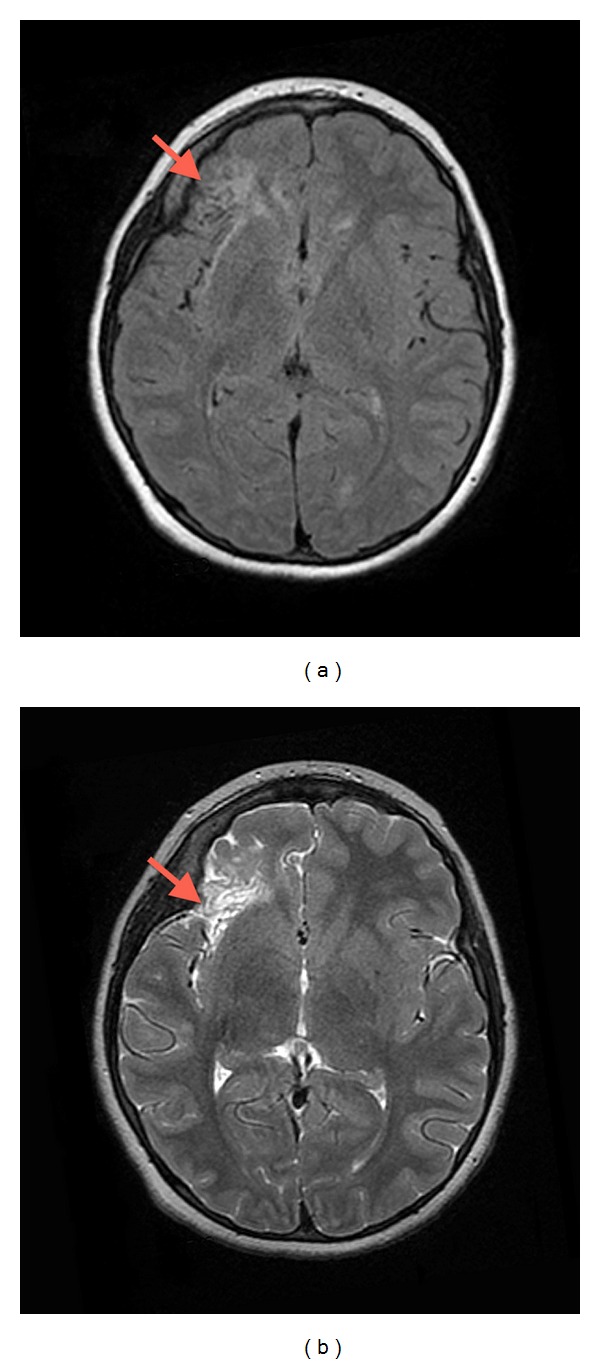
Magnetic resonance imaging studies reveal an abnormality in the right frontal operculum [(a) Axial T2 FRFSE; (b) Axial T2 FLAIR].
